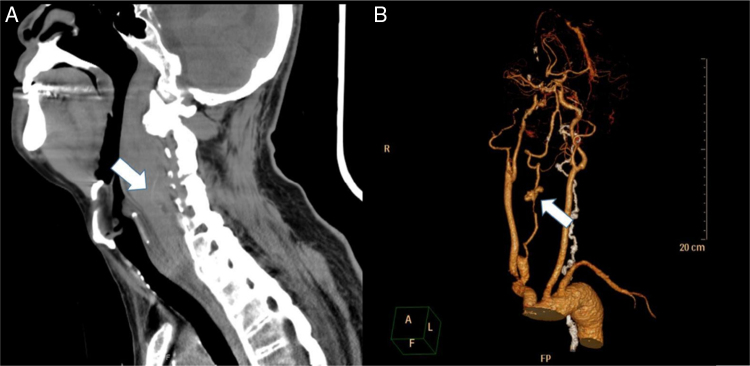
Contrast-enhanced computed tomography scan of the neck showing (A) the retropharyngeal hematoma extending from the base of the skull to the C7 level and syndesmophytes of the spine and (B) the pseudo-aneurysm formation on the right vertebral artery.
